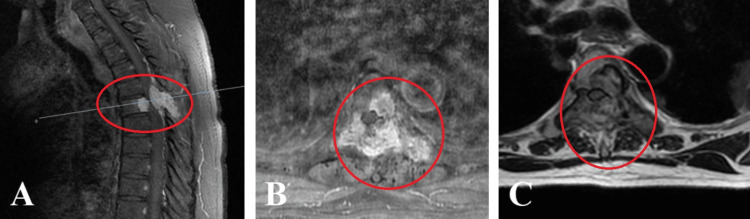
Sagittal (A) and axial (B, C) MRI scans of the thoracic spine show a metastatic tumor at T5, involving the vertebral body, a portion of the left posterior wall, both pedicles, laminae, and spinalis muscles, and invading the spinal canal with compression of the same. There is an absence of CSF passage.
